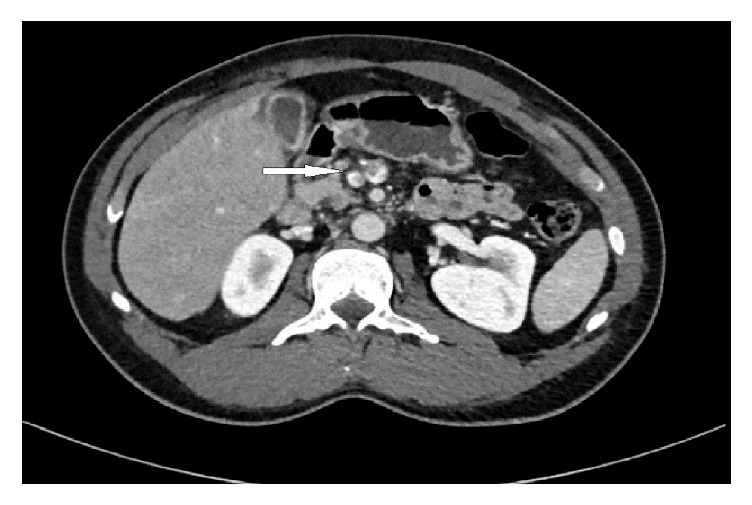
Contrast-enhanced CT scan showing pericholecystic fluid and partial filling defect in the superior mesenteric vein (white arrow).
